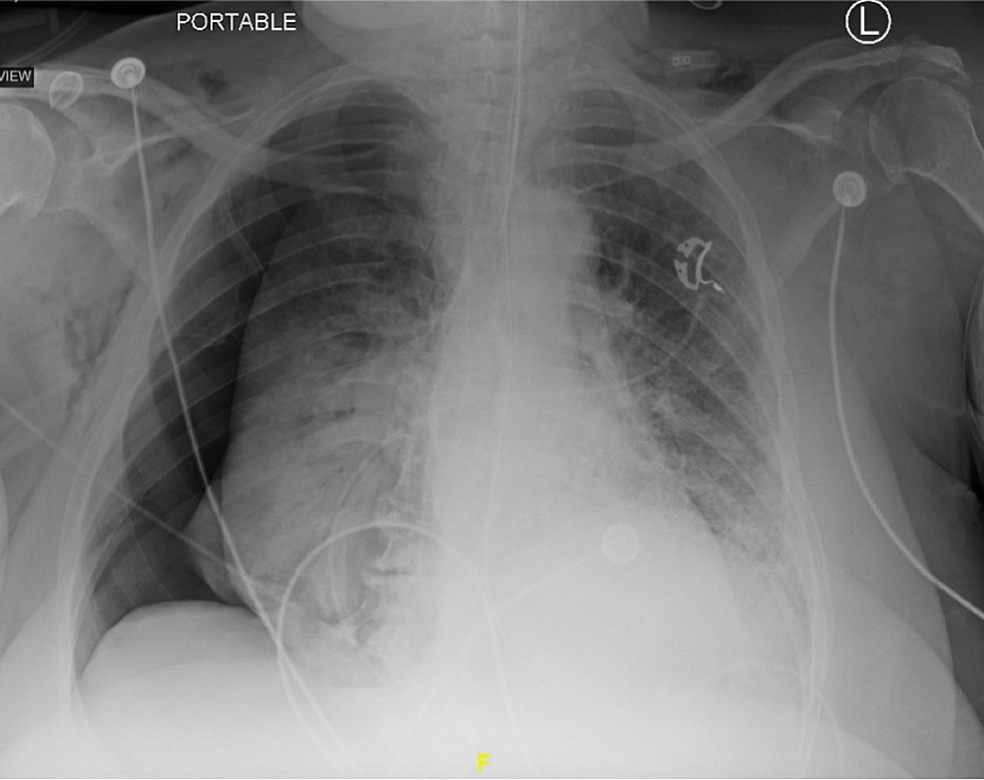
Chest radiograph of a large right-sided pneumothorax with leftward mediastinal shift requiring the placement of thoracostomy tubes
